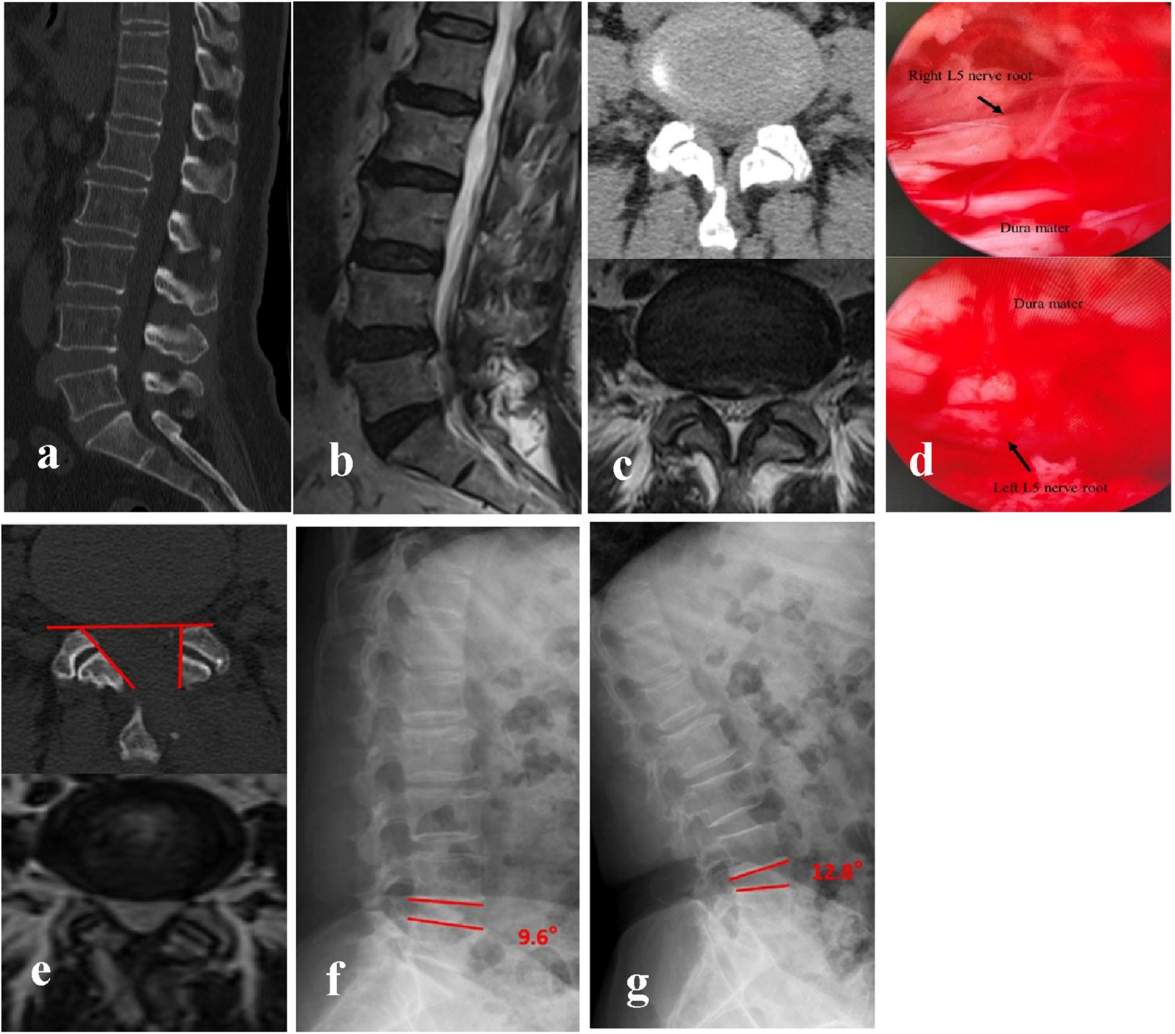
BEBD was performed via the left-sided approach for bilateral decompression at the L4/5 segment. (a–c) Preoperative CT and MRI demonstrated bilateral lateral recess stenosis at L4/5, resulting in significant compression of bilateral L5 nerve roots. (d) Intraoperative visualization confirmed adequate decompression and relaxation of bilateral L5 nerve roots following the procedure. (e) Postoperative CT revealed partial resection of the left medial FJ (FJPR: 73.4%) and satisfactory preservation of the right FJ (FJPR: 98.1%). Bilateral LR were sufficiently decompressed. MFSA measured 88.9° (ipsilateral) and 54.6° (contralateral). Postoperative MRI at 3 months after surgery documented a 98% increase in DSCAER, confirming sustained decompression. (f,g) Dynamic flexion-extension radiographic evaluation at final follow-up confirmed preserved segmental stability at the L4/5 level, with no evidence of abnormal motion or pathological listhesis observed.
